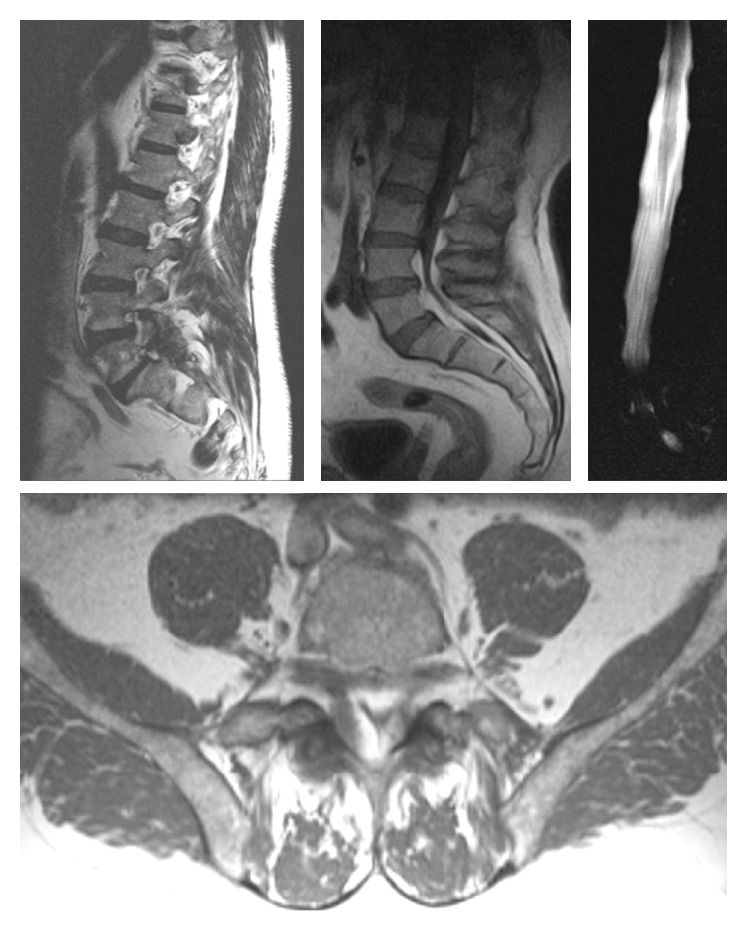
Preoperative MR. T1-weighted axial and sagittal image showed Y sign and thin dural sac. MR-myelogram shows stop at L4-S1 levels. Sagittal T2- (left) and T1- (right) weighted MR images showing Modic type I endplate change at the L4-L5 and L5-S1 levels consisting of low signal intensity on T1 images and high signal intensity on T2 images.
